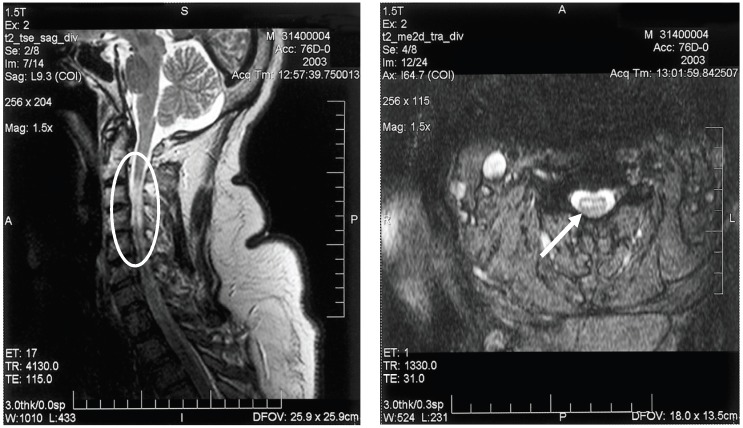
Sagittal (A) and axial (B) T2-weighted magnetic resonance imaging of the cervical spinal cord of a patient with bilateral upper extremity paralysis and respiratory failure from West Nile poliomyelitis, displaying the increased signal in the anterior spinal cord (circle and arrow).
